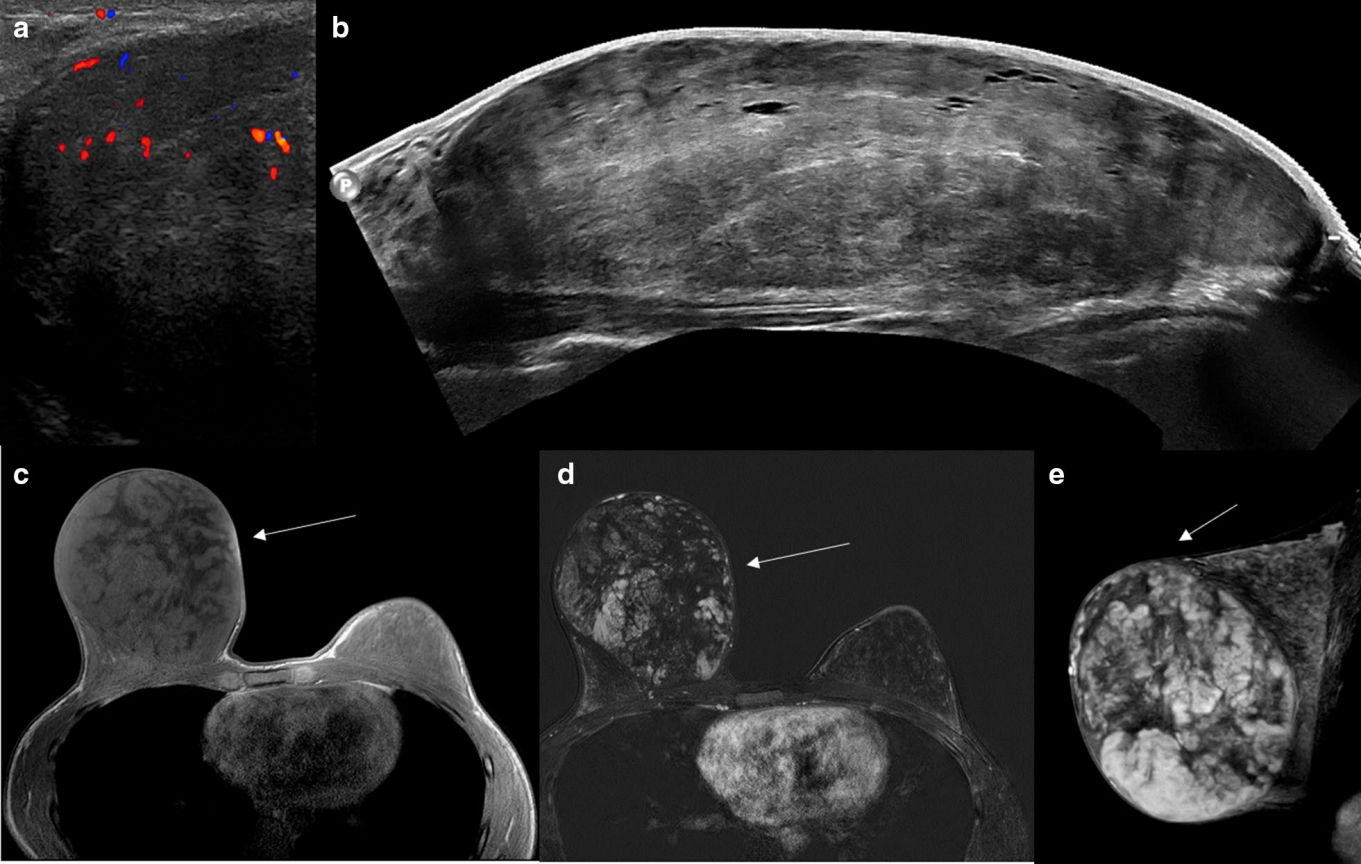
Hamartoma. A 14-year-old presented with unilateral breast enlargement. A mass was not palpable on examination. Color Doppler (a) and longitudinal panoramic ultrasound grayscale images (b) show a hypervascular, 17-cm isoechoic breast mass replacing the entire breast. T1-weighted non-fat-suppressed (c) and T1-weighted fat-suppressed (d) post-contrast MRI images show a well-circumscribed, heterogeneously enhancing breast mass suspicious for a sarcoma (arrow). T2-weighted sagittal images (e) show moderate T2 signal with marked heterogeneity (arrow). Pathology at the time of surgical excision showed hamartoma with areas of pseudoangiomatous stromal hyperplasia and fibroadenomatoid change
